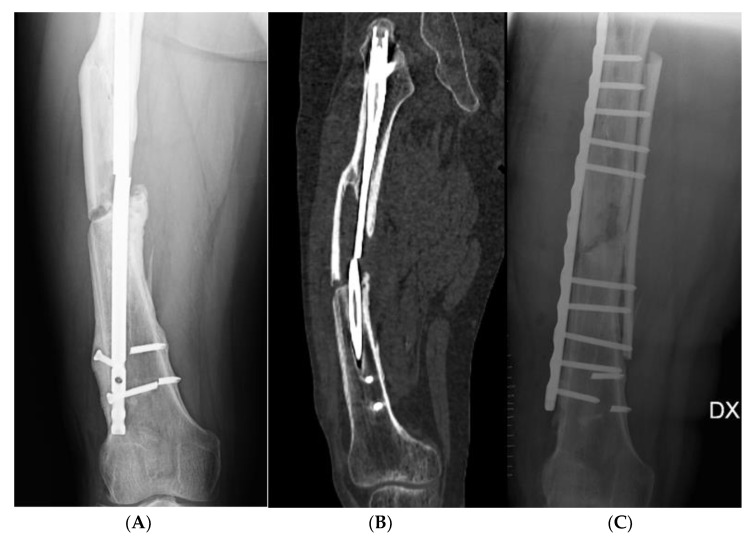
46-year-old patient with a closed 32-B2 fracture. (A) Anteroposterior X-ray showing PSA at the middle third of femoral diaphysis, initially treated with an intramedullary nail, which appear broken at the fracture site. (B) Coronal CT section of the femur showing the PSA focus, the broken nail and posterior bone loss. (C) Anteroposterior X-ray of the femur after revision surgery with lateral plate and screws and medial bone graft splint.
